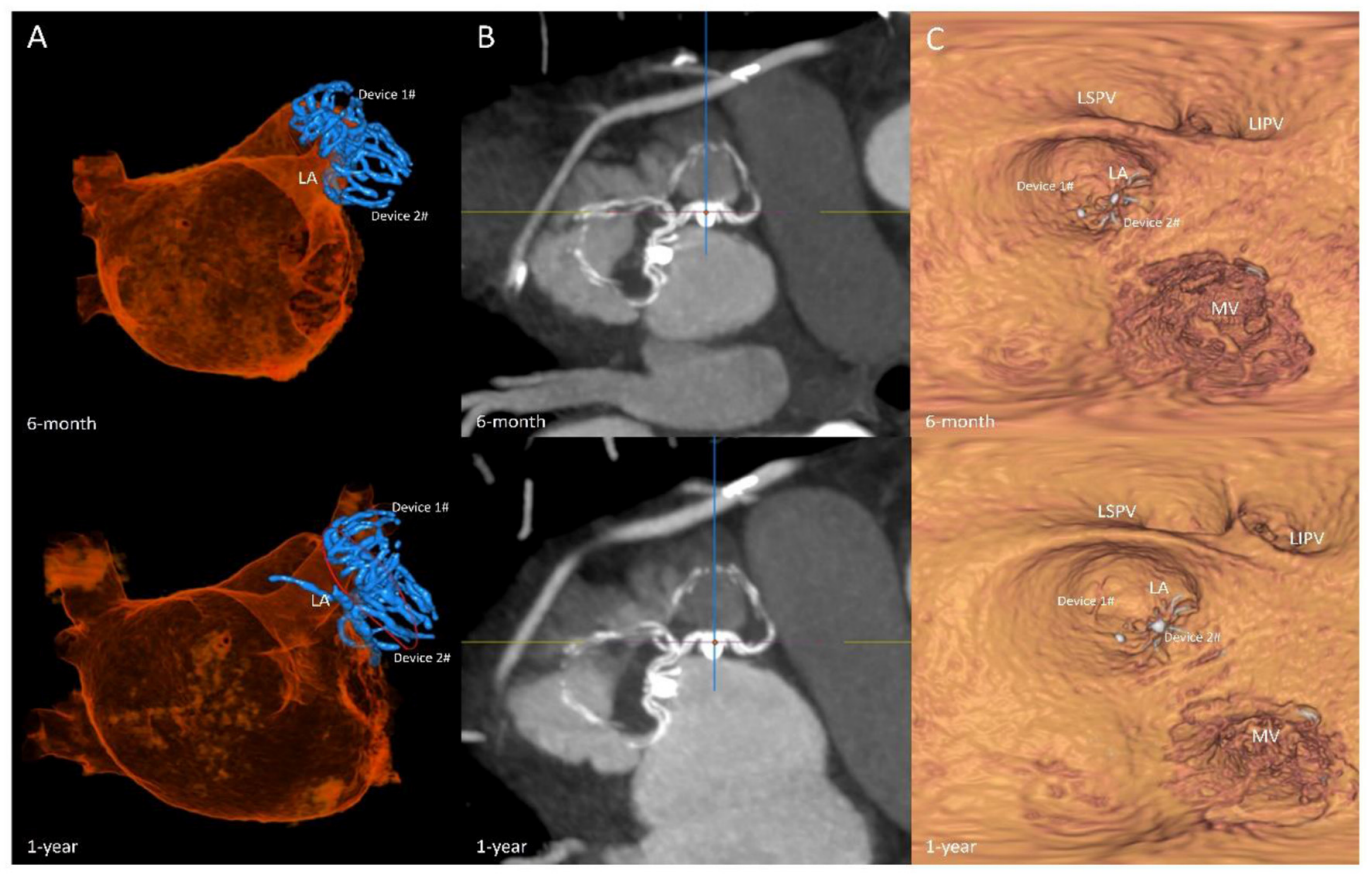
Follow-up imaging. Multiplanar reconstruction evaluating the anatomic position (A); volume-rendering CT image evaluating the residual shunt after surgery (B); multiplanar reconstruction evaluating the endothelialization of the occlusions (C).
